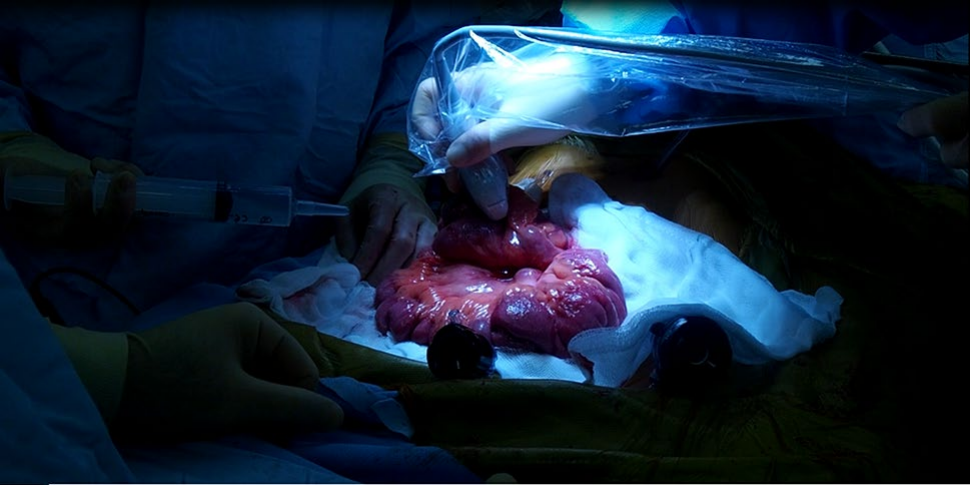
Intraoperative ultrasound scan
